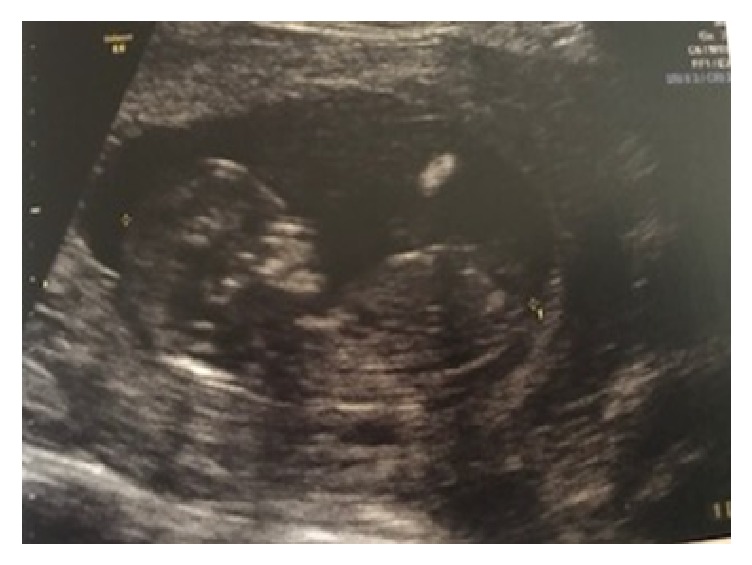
Ultrasound showing topic pregnancy at 13 weeks of gestation.
